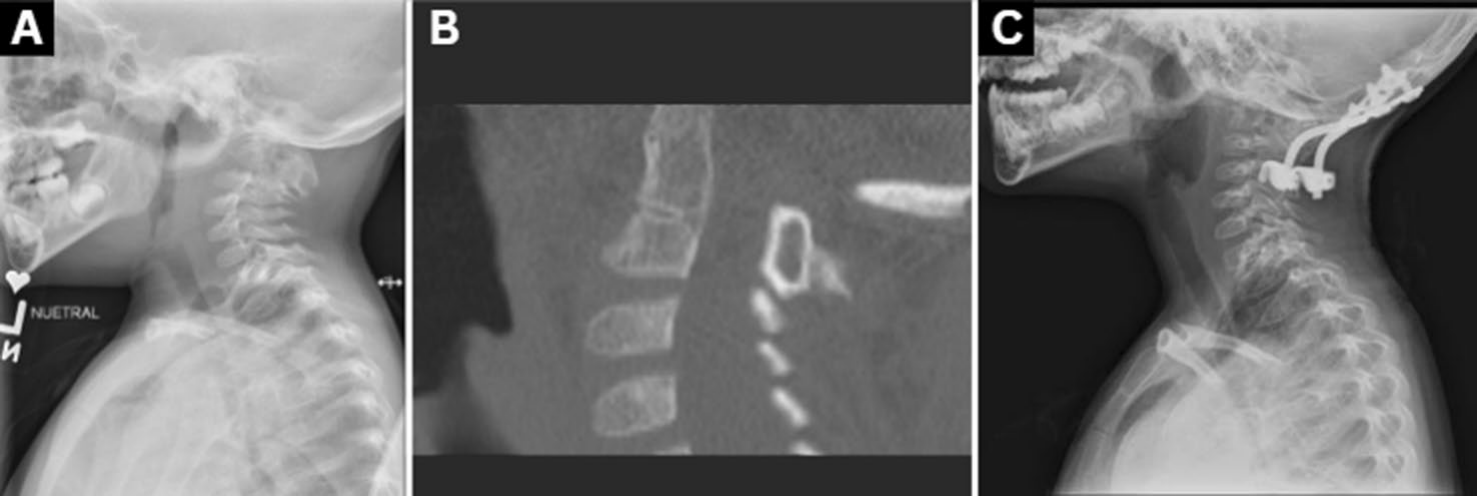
A Four year-old male who had 3 previous Chiari decompressions and developed basilar invagination. B He initially underwent an attempt at an uninstrumented occipitocervical fusion but developed a nonunion confirmed on CT scan with significant resorption of the graft. C This was revised to an instrumented fusion and radiographs with 2 years follow-up confirming an abundant fusion mass
